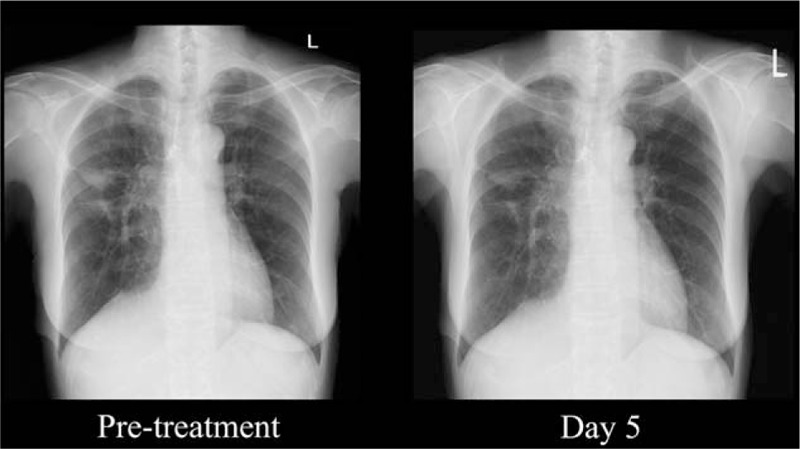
Chest x-rays of case 1. Shrinkage of the right pulmonary nodule after 5 days osimertinib administration.
